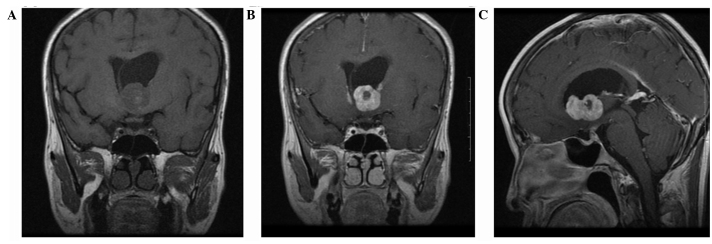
MRI observation of the solid mass in the anterior third ventricle. (A) Non-contrast-enhanced coronal T1-weighted MRI showing low signal intensity of the solid mass in the anterior third ventricle, which infiltrated into the lateral ventricle. (B) Contrast-enhanced coronal T1-weighted MRI and (C) contrast-enhanced sagittal T1-weighted MRI showing strong heterogenous enhancement with a non-enhanced hemorrhage. MRI, magnetic resonance imaging.
